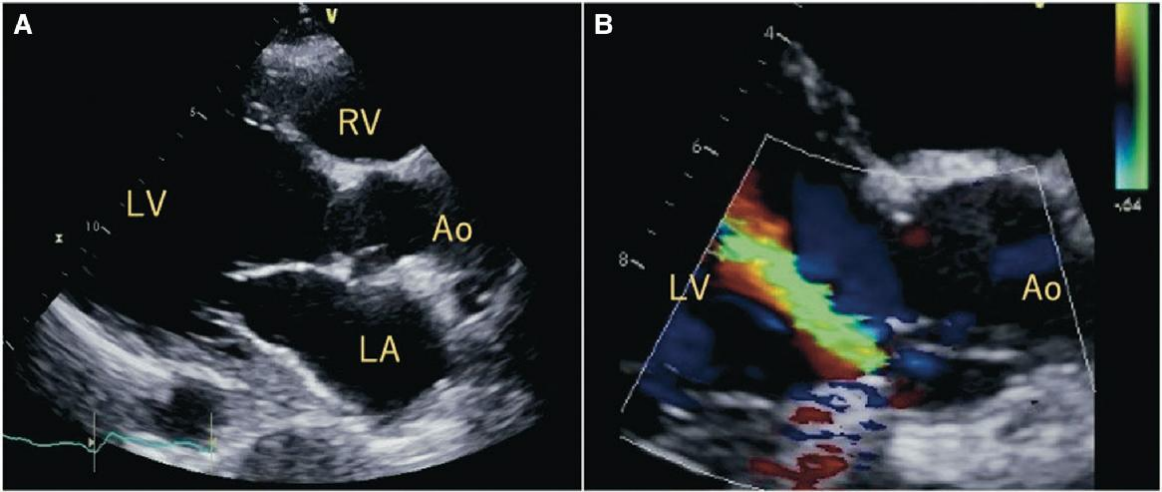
Pre-operative transthoracic echocardiography. (*A*) The left ventricle is dilated. There is no vegetation. (*B*) Severe aortic valve regurgitation is detected. Ao, aorta; RV, right ventricle; LA, left atrium; LV, left ventricle.
